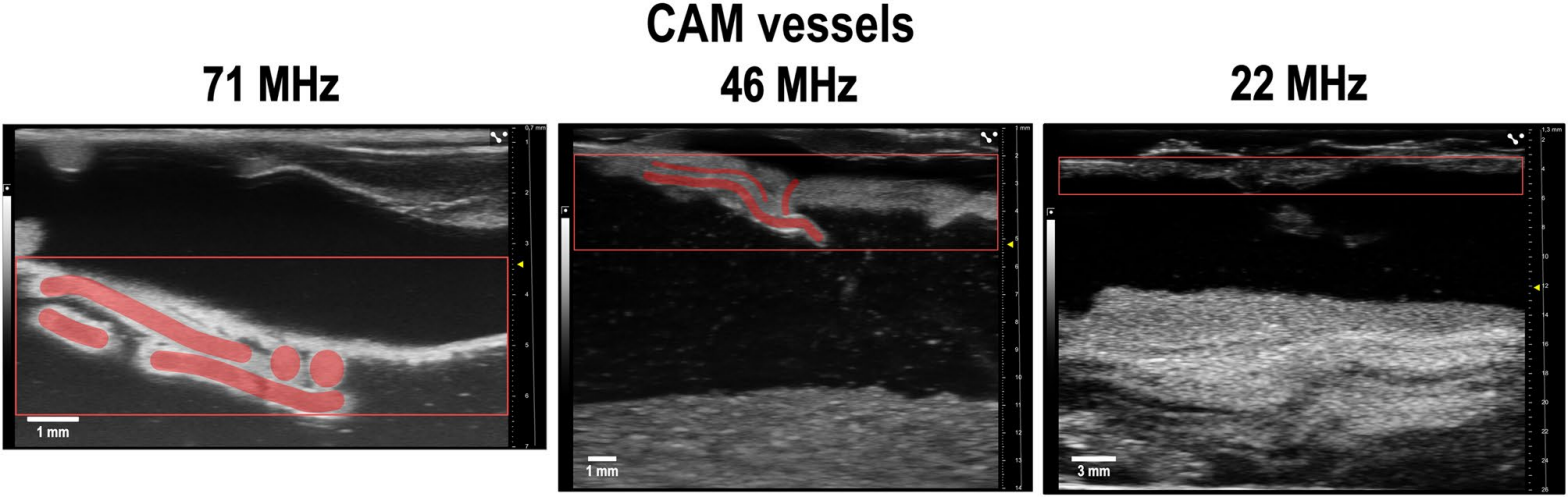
Transducer comparison for imaging of the CAM vasculature. Ultrasound images of CAM vessels were acquired with three ultrasound transducers of different frequency ranges (Supplementary Videos 9–11): UHF70 transducer at a frequency of 71 MHz (central frequency 50 MHz), UHF48 transducer at a frequency of 46 MHz (central frequency 30 MHz) and UHF22 transducer at a frequency of 22 MHz (central frequency 15 MHz). The region of interest is marked with a red box and CAM vessels are highlighted in red.
